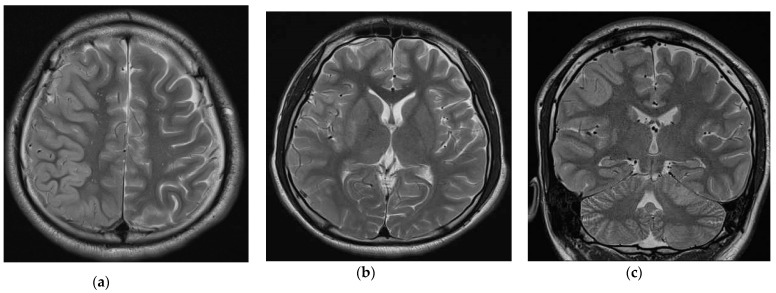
Brain MRI for individual 9 at day 15 of disease onset. (a,b) The axial brain MRI (TE 103 ms; TR 3820 ms) shows diffuse cortical swelling over the right frontal and temporal areas and T2WI high signal intensity over the right putamen and right thalamus. (c) The coronal MRI (TE 83 ms; TR 4000 ms) shows T2WI high signal intensity over the right putamen, right thalamus, and left cerebellum.
